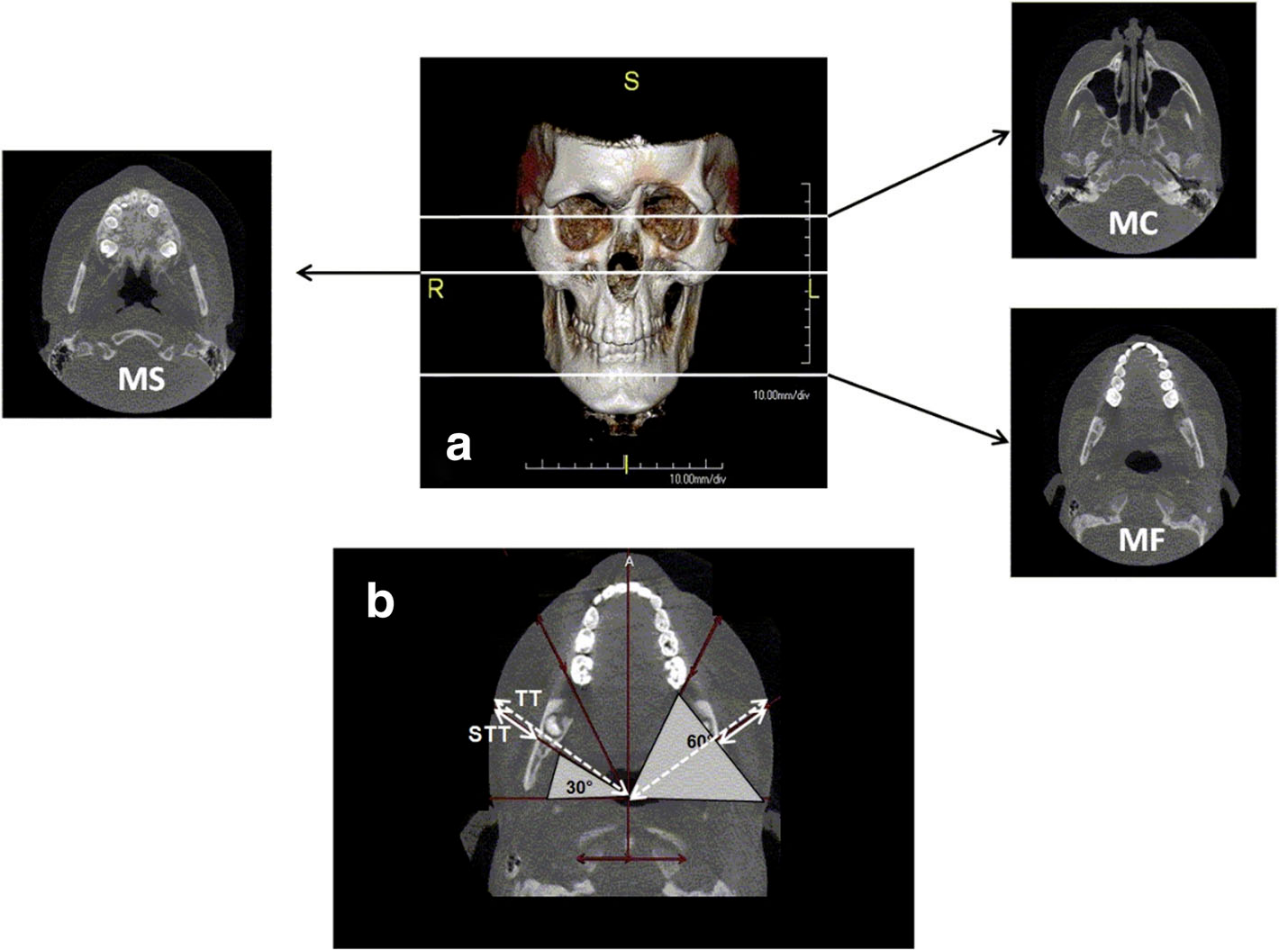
a 3D–Cone Beam Computed Tomography (CBCT) showing the levels of the three transverse sections (mandibular condyle, MC, maxillary sinus MS, mandibular foramen MF) selected for the comparison of affected and unaffected side. b Example of measurement of total thickness (TT) and soft tissue thickness (STT) at 30° and 60° of the MF section in both affected and unaffected side of the face
